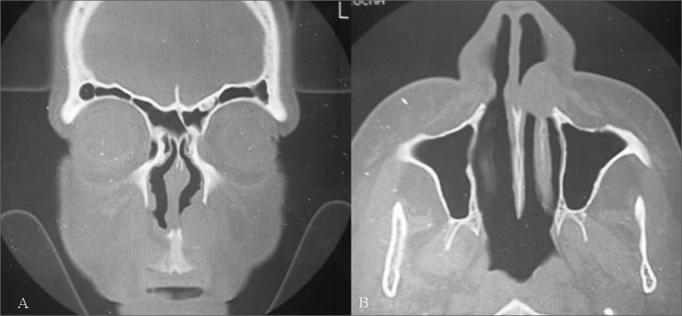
Radiological findings (computed tomography) of a nasolabial cyst – coronal section (A) and axial section (B) of a left unilateral cyst.
